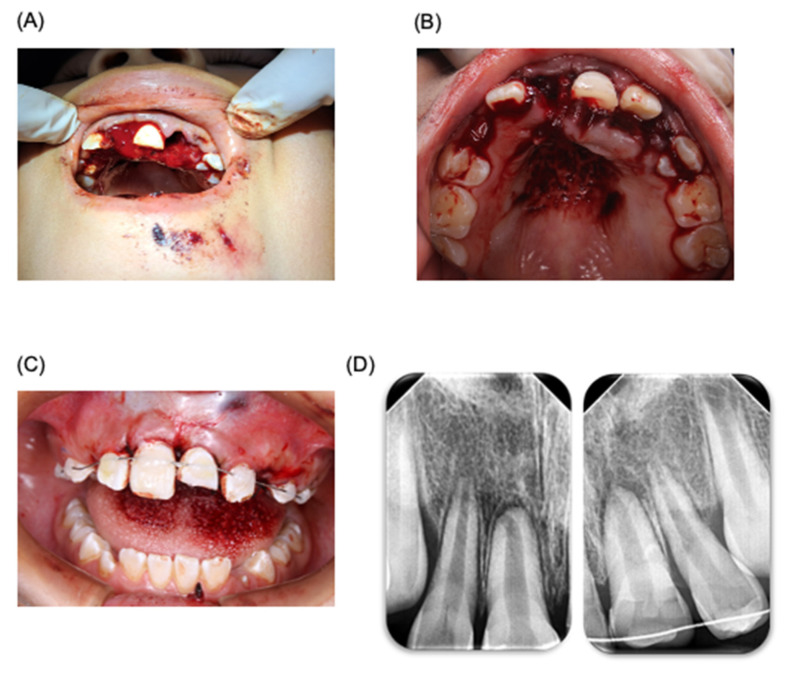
Odontology treatment of patient. Management of dentoalveolar trauma in pediatric patient. (A) Photograph of dentoalveolar trauma in pediatric patient. (B) Reimplantation of tooth 22. (C) Management of trauma using a wire–resin splinting technique. (D) Periapical X-ray after splinting treatment.
